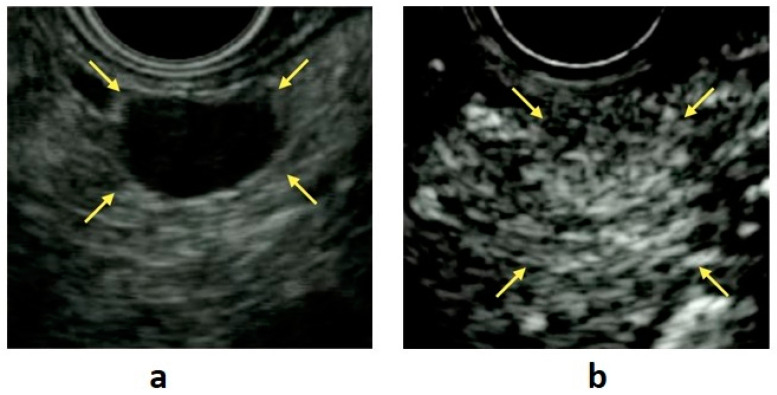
(a) B-mode endoscopic ultrasound (EUS): a circular hypoechoic mass is seen in the body of the pancreas (yellow arrow). (b) Contrast-enhanced EUS: the mass shows early enhancement compared with the surrounding pancreatic parenchyma (yellow arrow).
